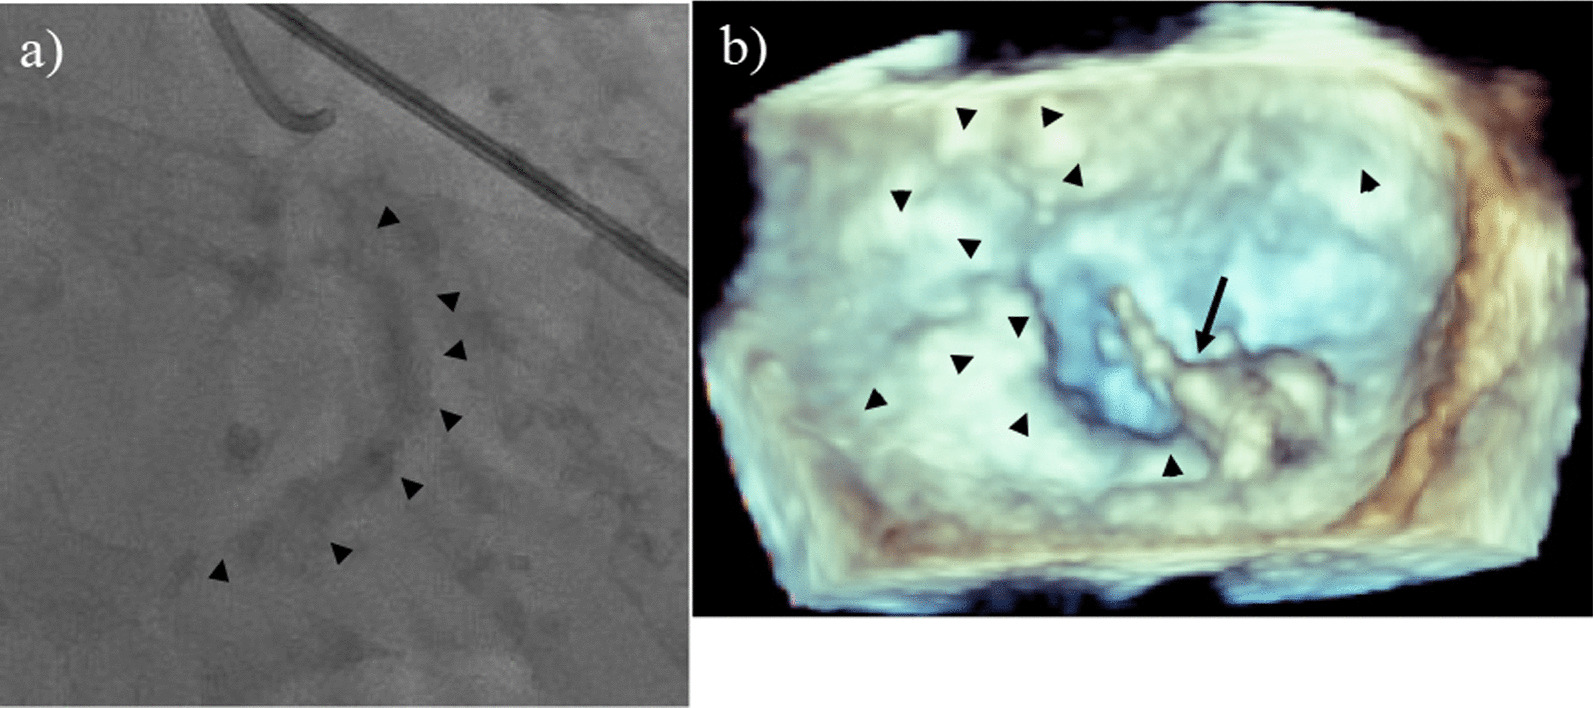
Preoperative cardiac fluoroscopy and 3-dimensional transesophageal echocardiography. a Preoperative cardiac fluoroscopy shows calcification (black arrowheads) around the posterior mitral annulus. b Preoperative 3-dimensional transesophageal echocardiography demonstrates extensive calcification of the mitral anterior and posterior annuli (black arrowheads) and prolapse and torn chordae of the posteromedial scallop of the posterior leaflet (black arrow)
